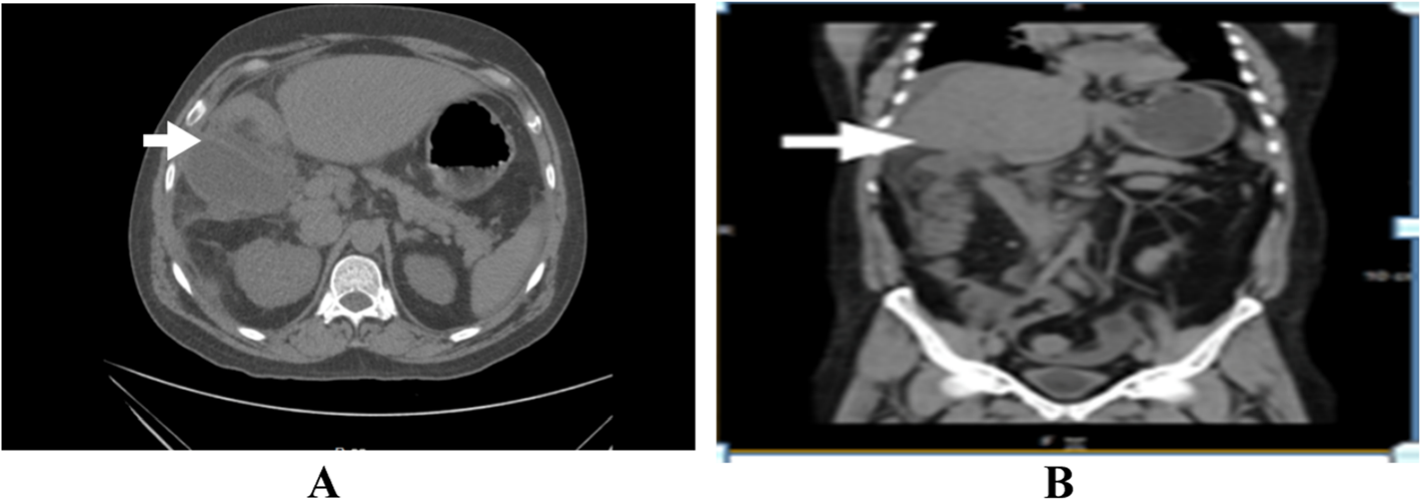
a, b:Axial and coronal CT scan of abdomen showing gall bladder wall edema with intense pericholecystic fat stranding (white arrow), free fluid around liver and showing edematous thickening (white arrow) at hepatic flexure
